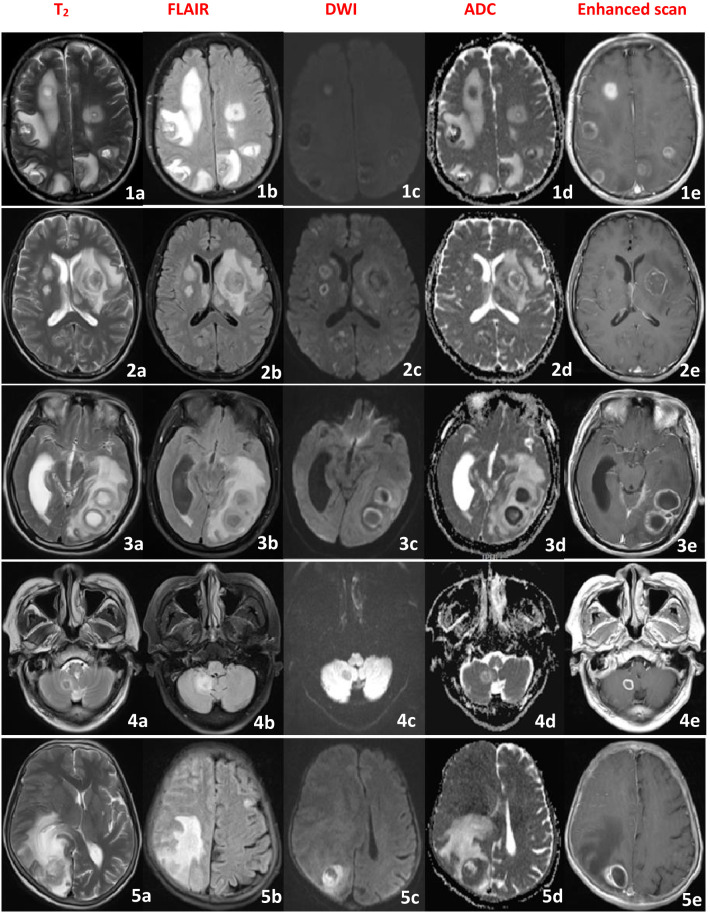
Brain MRI image of the patient (1-5), showing multiple intracranial circular lesions, High signal in the T2 and FLAIR sequence, low signal in the DWI and ADC in the center of the lesion, high signal around the lesion, and irregular circular enhancement in the enhanced scan.
